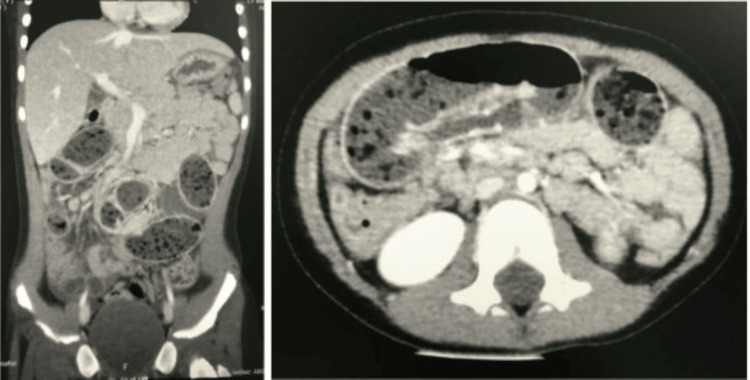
On readmission, CT abdomen (coronal and axial view) shows dilated proximal small bowel and collapsed distal bowel indicating intestinal obstruction.
